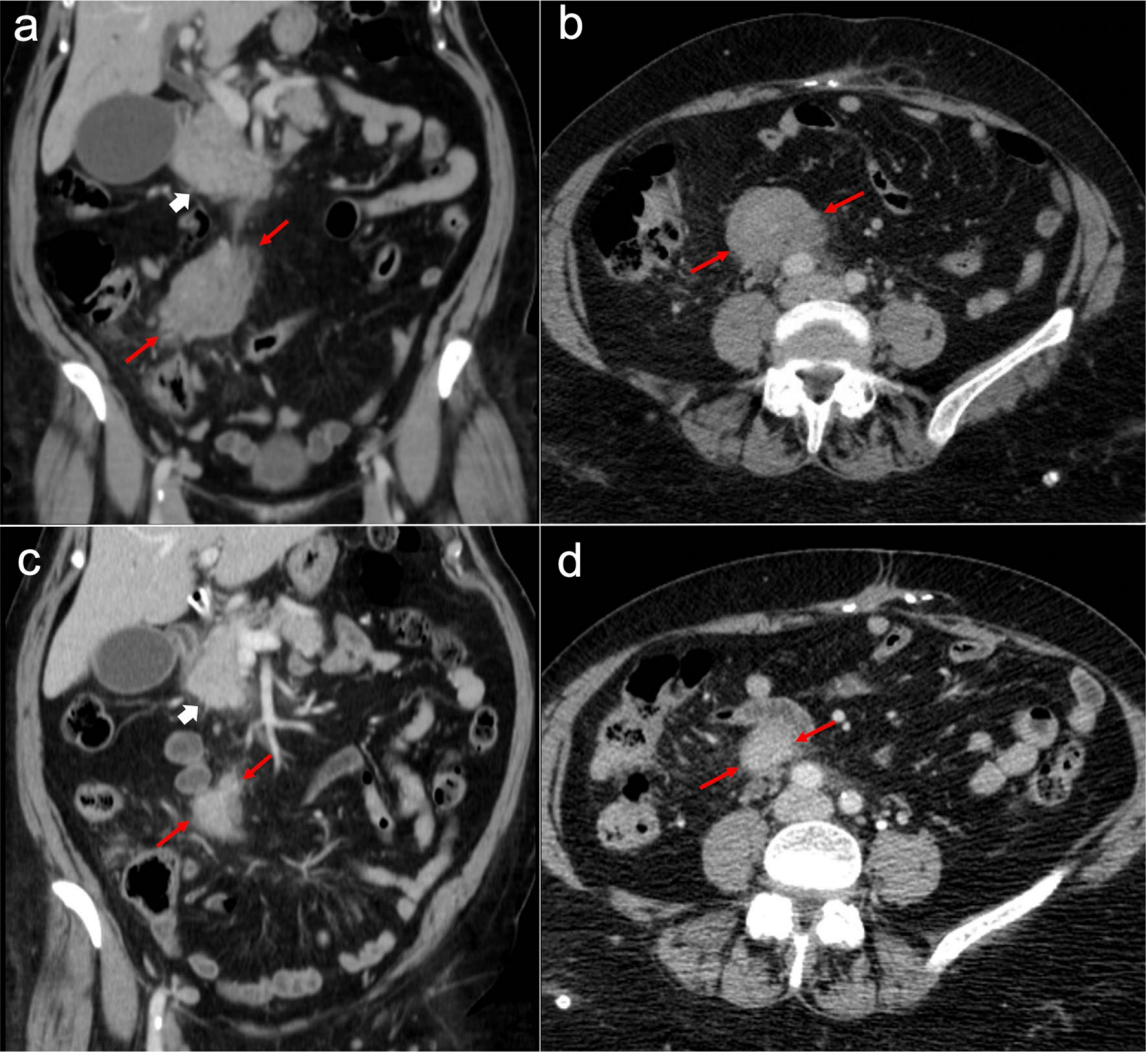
Mesenteric inflammatory pseudotumor in a 73-year-old woman with history of autoimmune pancreatitis and IgG4-related sclerosing cholangitis. Axial (a) and coronal (b) CT images in the portal phase show an increase in size of the pancreatic head secondary to autoimmune pancreatitis (white arrow) and a solid pseudonodular mass in the small bowel mesentery (red arrows). c, d Follow-up CT scan performed one month after steroids treatment shows a dramatic response, with a significant reduction in both the pancreatic head (white arrow) and the mesenteric mass (red arrows)
